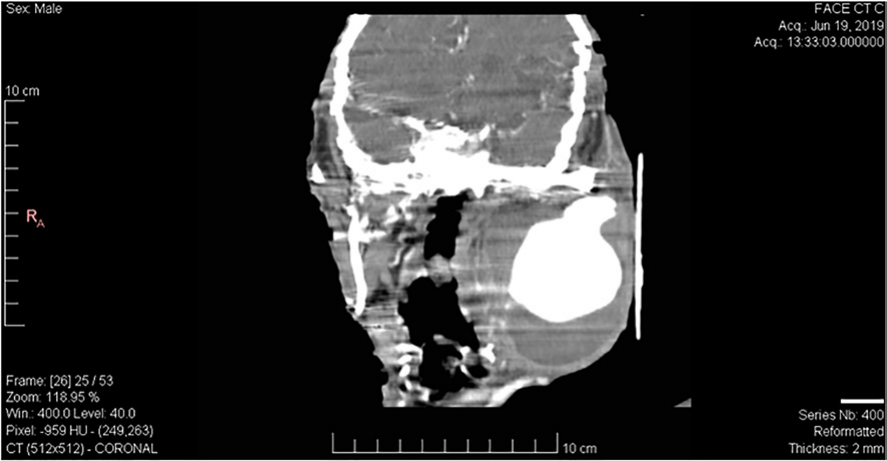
CT angiography shows a large lesion in left side of neck from left external carotid artery supplying the enhancing mandibular arteriovenous fistula
